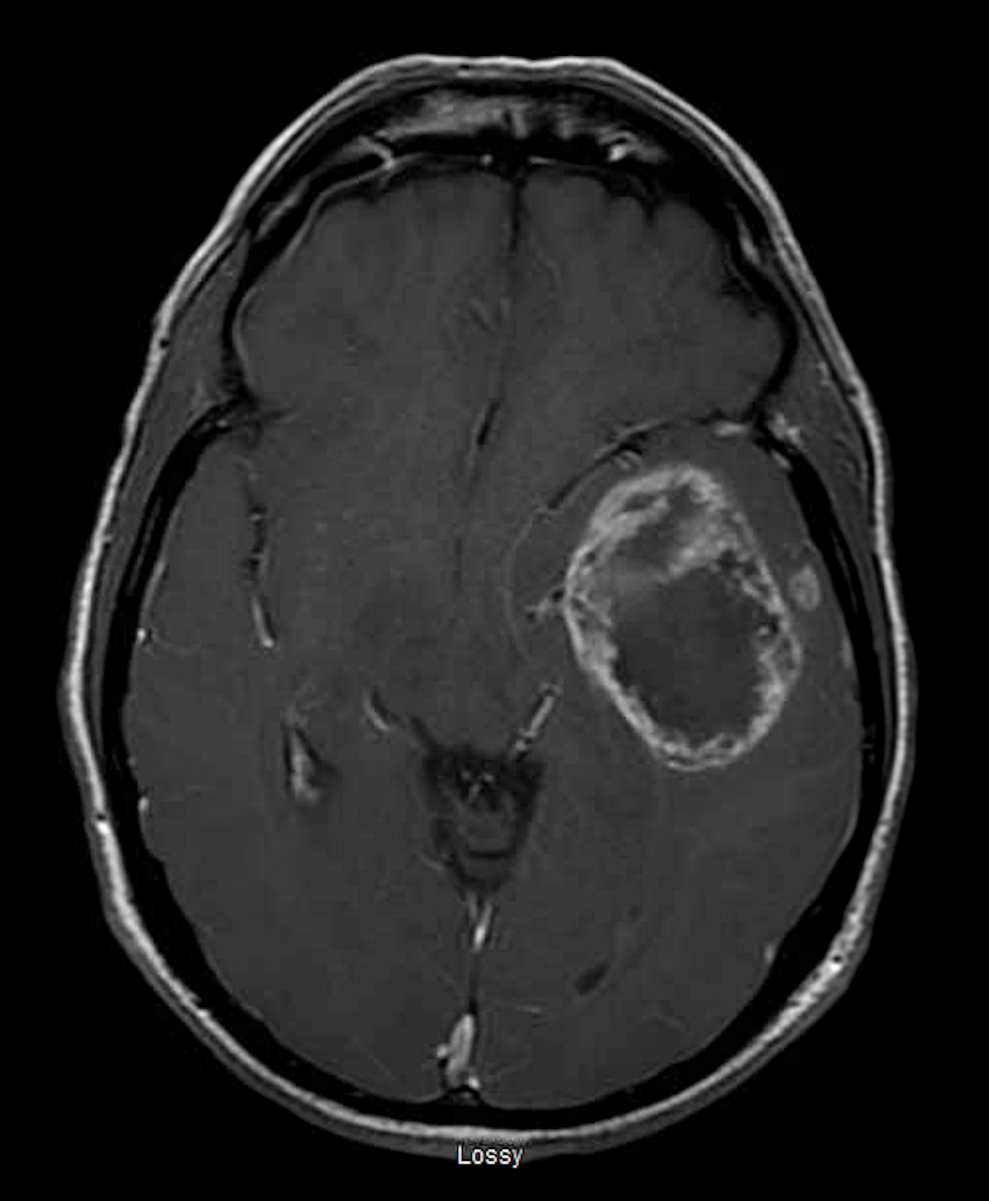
Brain MRI, Axial View with Contrast Enhancement
